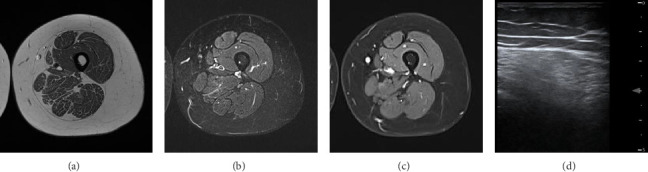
MRI of the thigh muscles in the transverse plane in (a) T1, (b) T2-TRIM and (c) Dixon with contrast agent. (d) Transversal sonography of the M. rectus femoris, vastus lateralis, right, revealing hyperechogenicity (modified Heckmatt Scale [5]: 2/4).
